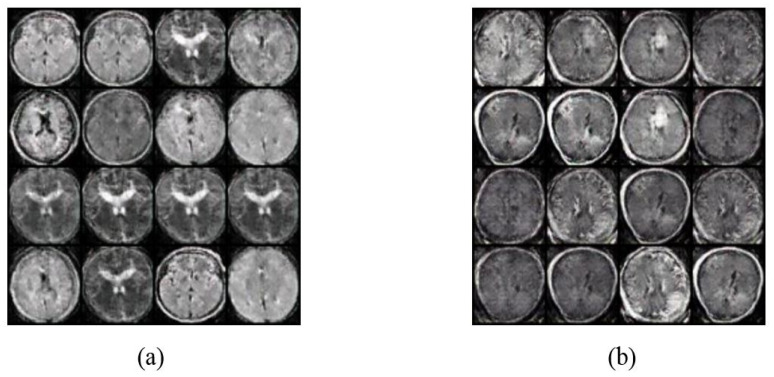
MRI scan images that are generated by applying DCGANs for (a) DCGANs no tumor images, and (b) DCGANs tumor images.
